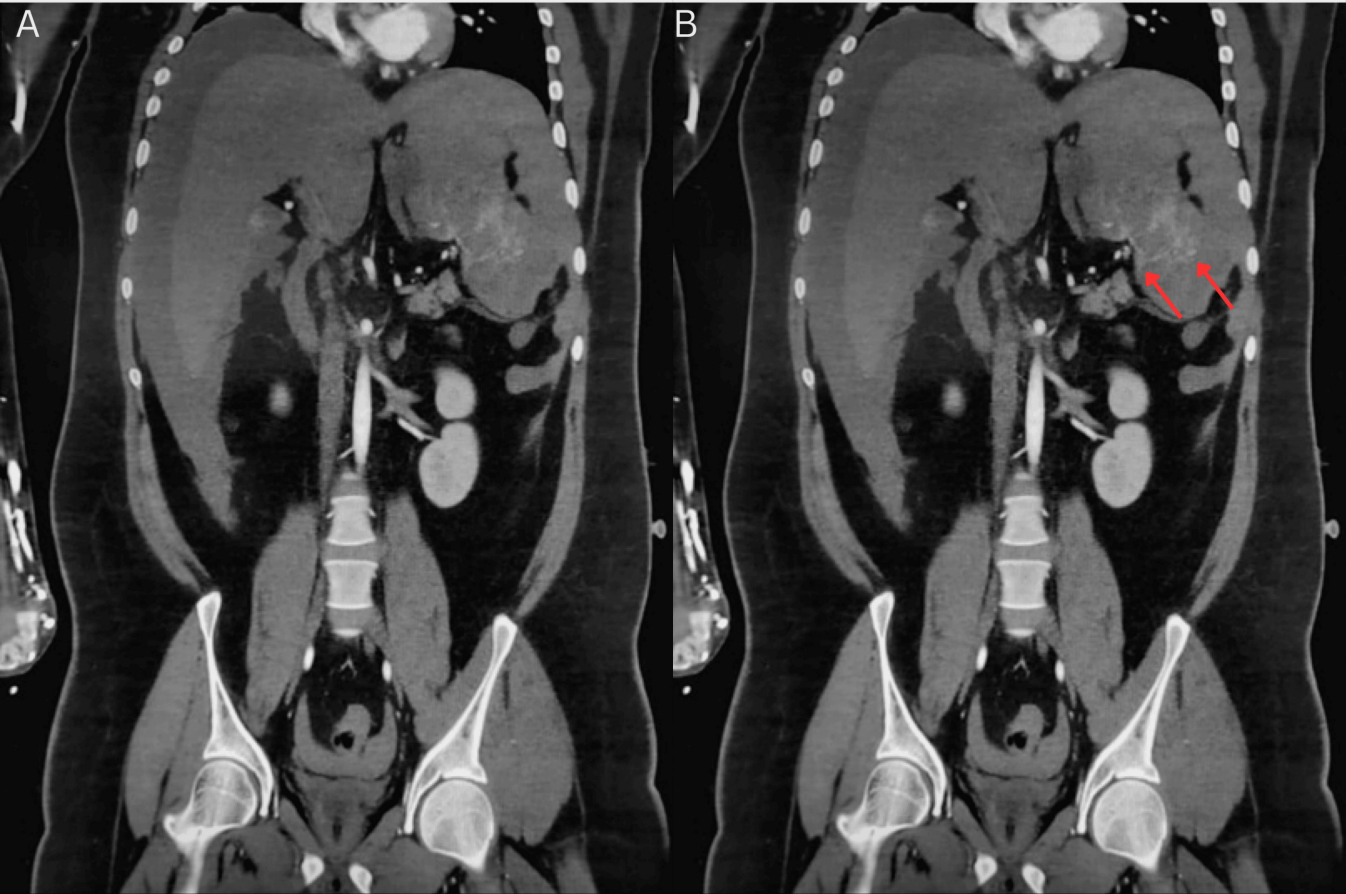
Contrast-enhanced coronal arterial-phase CT of abdomen demonstrating splenic rupture Pre-operative coronal arterial-phase contrast-enhanced CT suggestive of splenic parenchyma with focal areas of contrast extravasation (red arrows). (A) shows the unannotated scan and (B) shows the annotated scan.
